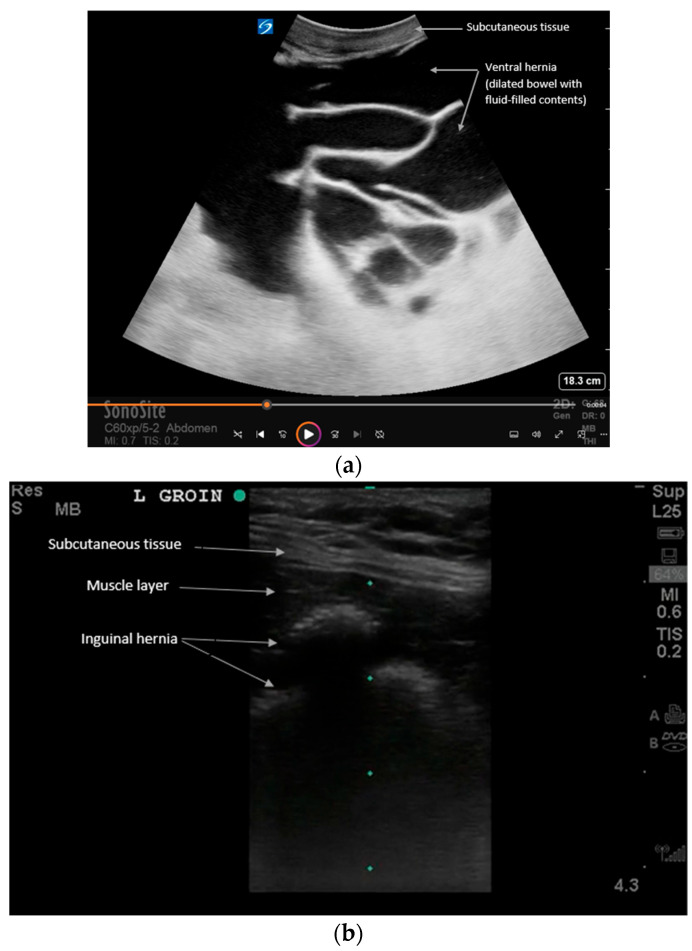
Examples of (a) ventral and (b) inguinal hernias that contain loops of bowel on POCUS. See Supplementary Videos S2 and S3 for examples of dynamic findings. Intestinal hernias can be evaluated with ultrasound at the bedside for faster recognition and manual reduction. Ultrasound reveals loops of bowel traversing the abdominal wall muscles. Dynamic assessment with Valsalva is recommended per expert consensus to evaluate reducibility and complications [11]. For example, the patient can be asked to perform a Valsalva maneuver to exaggerate the hernia during real-time ultrasound. Increased vascular flow, extra-luminal fluid, bowel dilation, or wall thickening can all suggest an increased risk for hernia incarceration or strangulation, which requires expedited treatment. Also, the lack of peristalsis within the bowel is a sign of complication [18].
